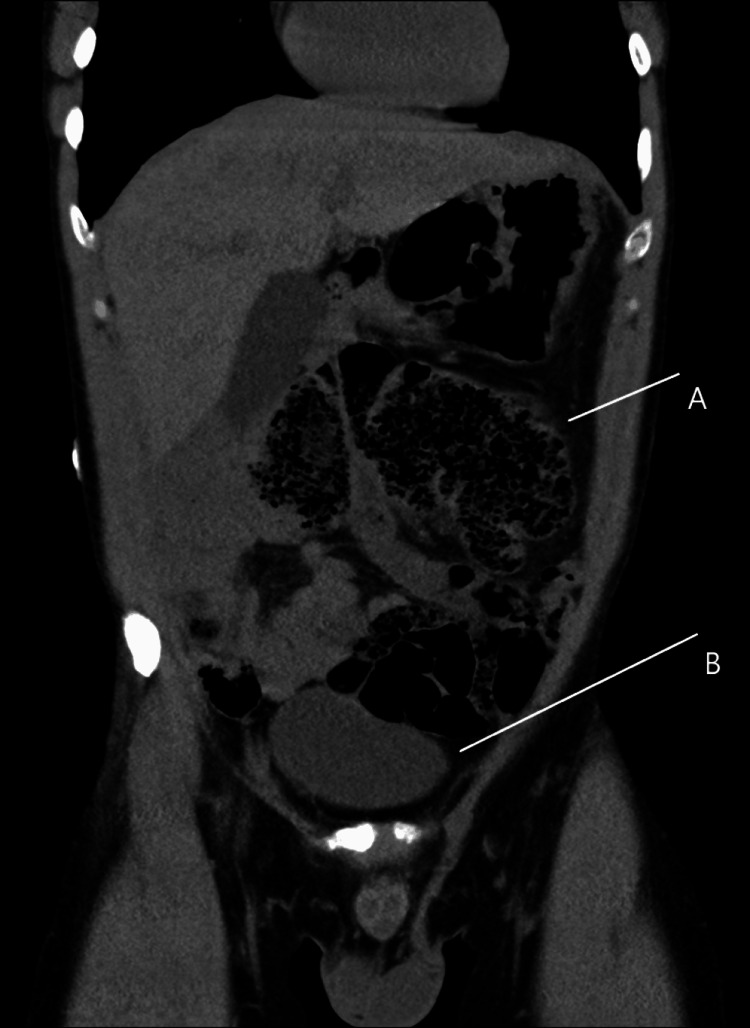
Abdominal CT, cross section, lower abdomen. Coronal section of an abdominal CT showing (A) abnormally positioned distended cecum in the mid- and left abdominal cavity and (B) displaced urinary bladder secondary to bowel distension.
